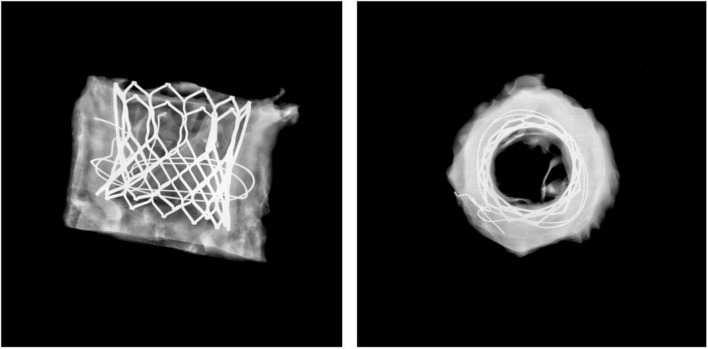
X-ray image. No deformations or damages in stent geometry.
